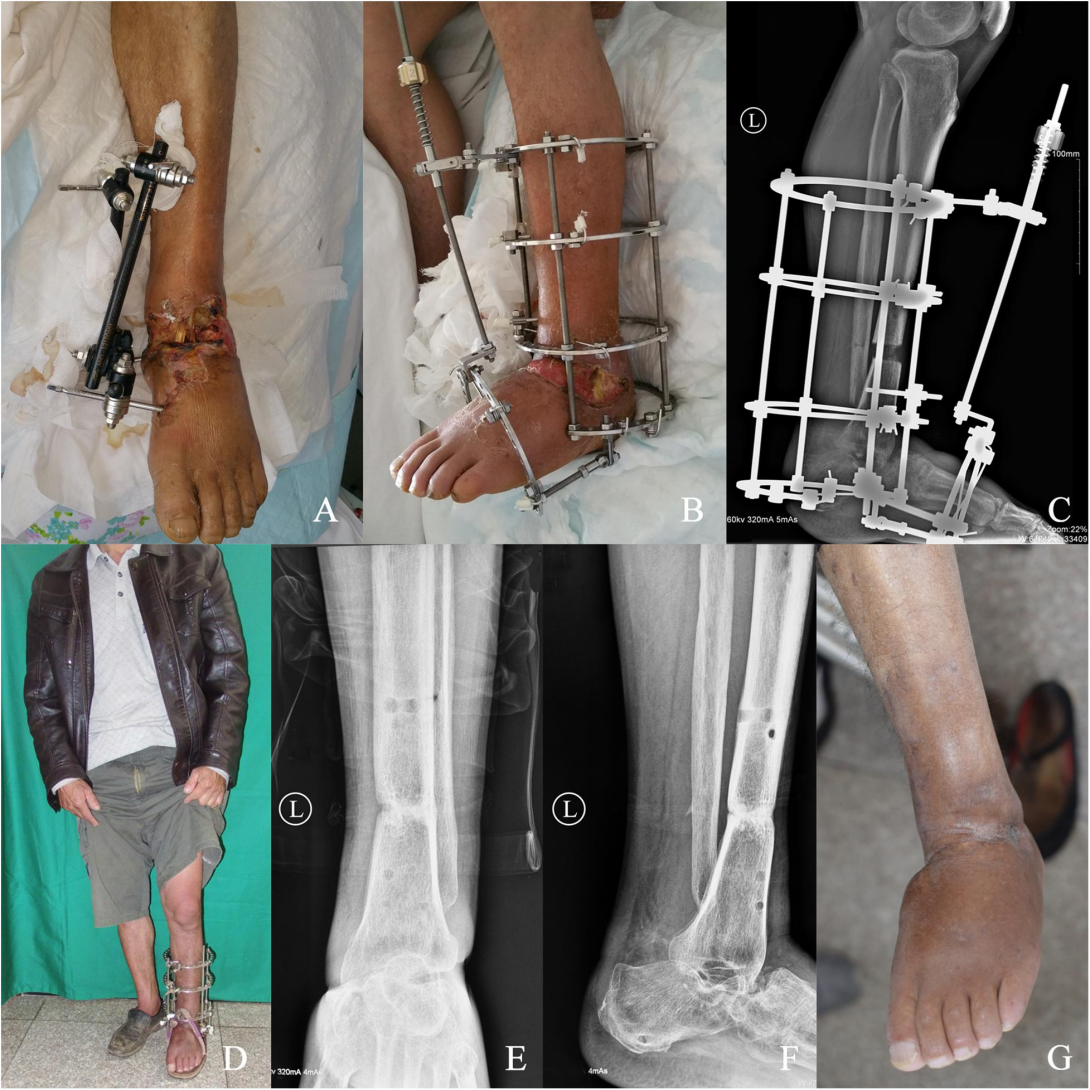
Male patient, aged 45 years: (A) preoperatively, diagnostic findings indicated the presence of infectious necrosis involving the talus, in conjunction with soft tissue infection, resulting in an initial AOFAS score of 27 points. (B) Following the exclusion of contraindications for surgical intervention, the patient underwent talus excision surgery and concomitant debridement of the affected skin soft tissues. Subsequent to meticulous wound care, a minimally invasive midshaft tibial osteotomy and tibio-calcaneal fusion procedure were meticulously executed. (C) Postoperative radiographic scrutiny showcased a mid-lower segment tibial transection, with the utilization of an Ilizarov external fixator in a state of bone traction. (D) Postoperative mobilization was initiated with the aid of crutches, complemented by a structured regimen of rehabilitation exercises. (E and F) A follow-up examination at two and a half months postoperatively revealed suboptimal tibio-calcaneal fusion, culminating in the formation of pseudoarthrosis. Noteworthy bone mineralization in the midshaft of the tibia necessitated the removal of the external fixator. (G) An extensive 1.8-year postoperative follow-up evaluation revealed the patient's satisfactory lower limb mobility and sensory function, with the pseudoarthrosis exhibiting no discernible impact on routine ambulation or physical activities, yielding an AOFAS score of 77 points.
